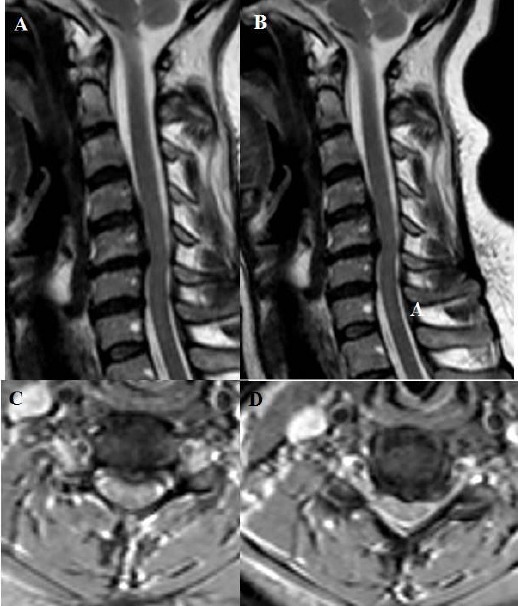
A, B, C, D: T2 weighted sagittal and axial MRI showing Compression to the medulla spinalis at two levels which are between cervical 3 and cervical 4, and between cervical 4 and cervical 5. Osteophyte formation and posterior longitudinal ligament ossification lead to this compression on medulla spinalis. Besides, cervical lordosis angle was measured 13.6 degrees on lateral X-ray at the preoperative period*.
